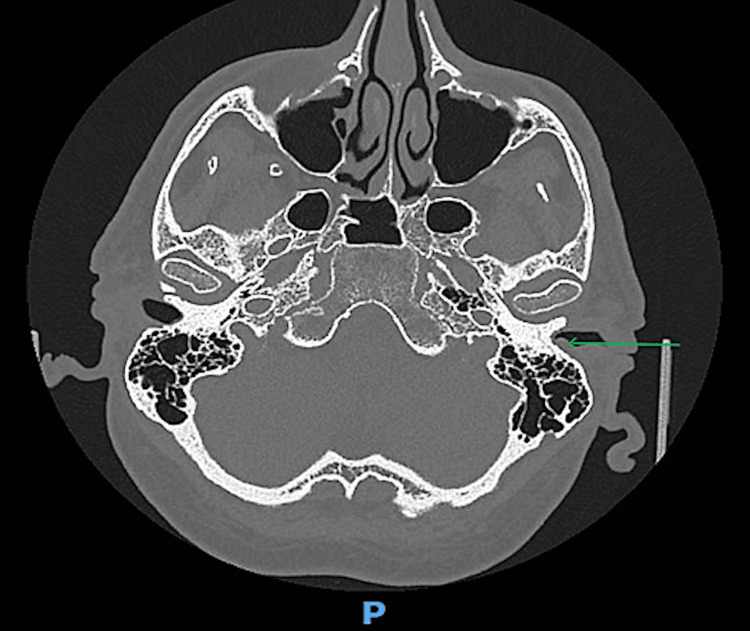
CT scan (axial view) of the temporal bone showing a 6 × 6 mm soft tissue lesion (green arrow) in the left external auditory canal without evidence of bony erosion
